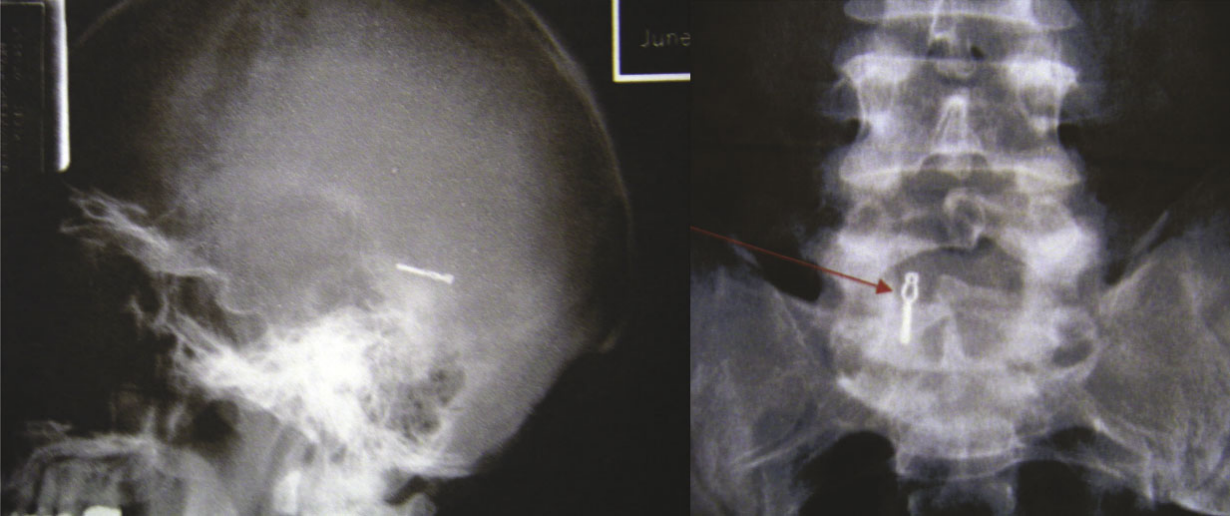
Imaging performed five years after AVM resection: a) Lateral skull film performed five years after surgery demonstrating displacement of one aneurysm clip and the absence of the other. b) Anterior posterior radiography of the lumbar spine revealing the location of the aneurysm clip in the lumbar spine (arrow).
